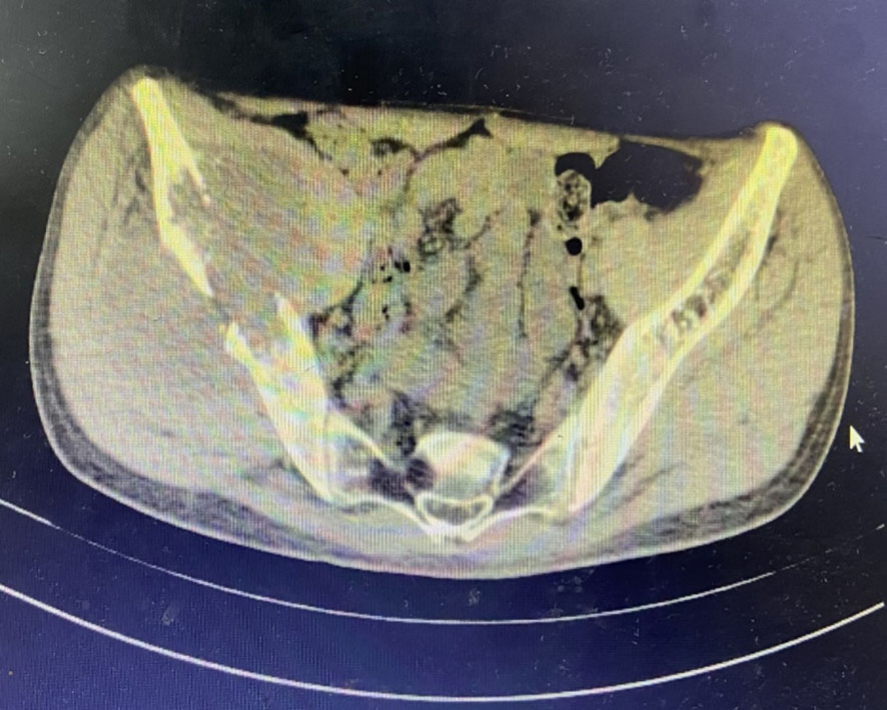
MRI imaging characteristics. Sacral and left iliac signal abnormalities.
